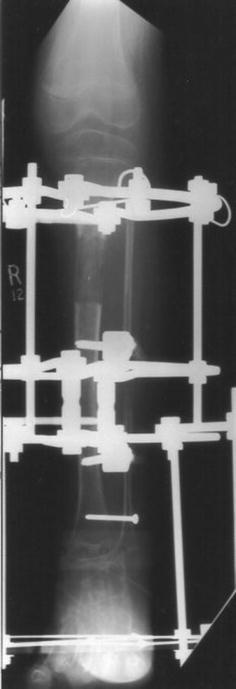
Radiograph AP view during lengthening
